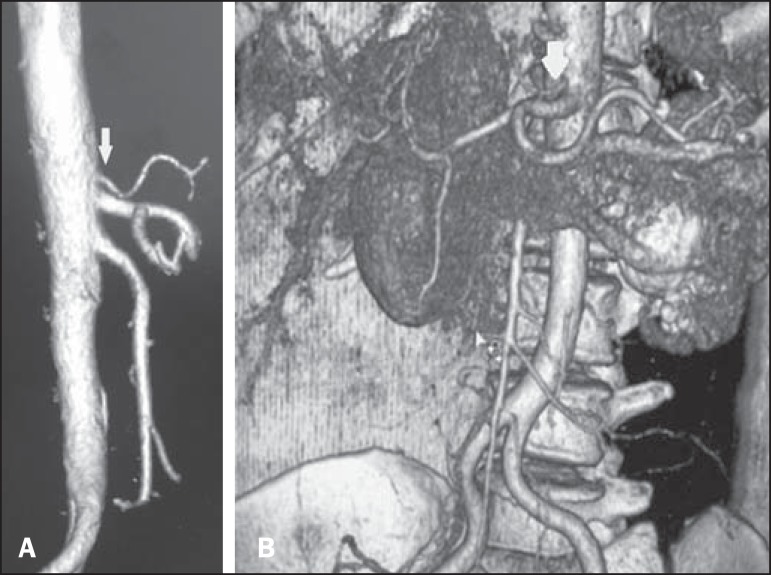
Computed tomography, coronal section with VR, demonstrating a
